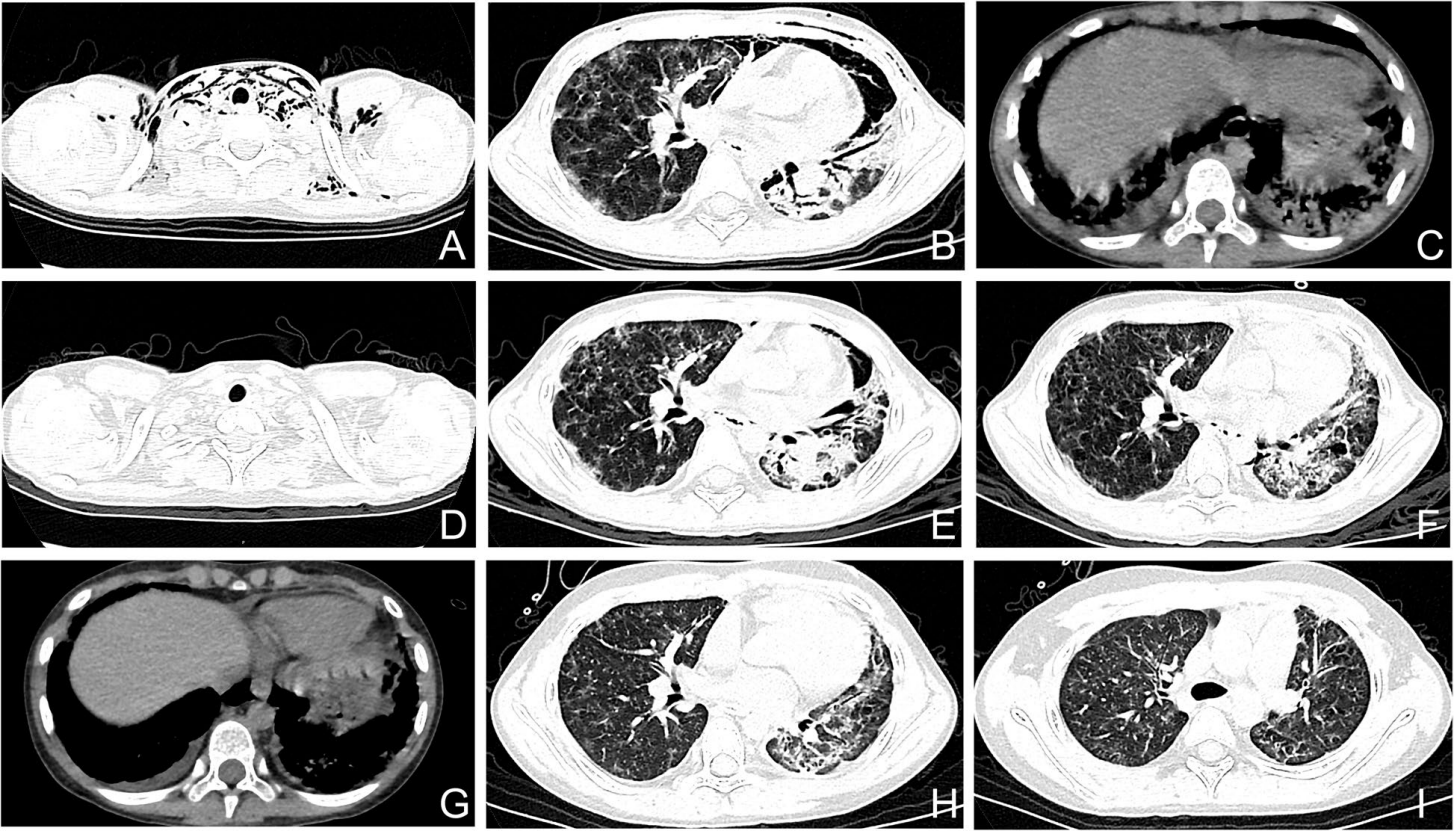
Radiologic findings of the patient. A-C On day 18 of illness, CT shows subcutaneous pneumatosis of the neck and bilateral chest wall, pneumomediastinum, consolidation, and reticular nodular shadow in both lungs, along with pericardial effusion and bilateral pleural effusion. D-E On day 23, subcutaneous air is essentially absorbed, pneumomediastinum is reduced compared with earlier, and consolidation and interstitial lesions in both lungs are present. F-G On day 30, pneumomediastinum is fully absorbed, and consolidation and pleural effusion are less pronounced than earlier. H-I One week after discharge, CT shows reticular nodular shadow, and both lungs exhibit residual consolidation, which have significantly resolved
